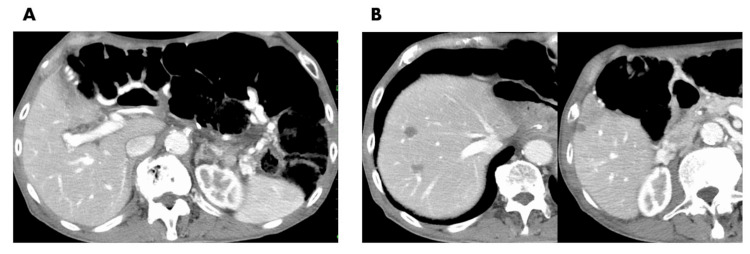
Computed tomography images after seven months of chemotherapy. (A) Shrunk pancreatic tumor and (B) shrunk coagulation area after microwave ablation with no evidence of local recurrence or emergence of new lesions.
